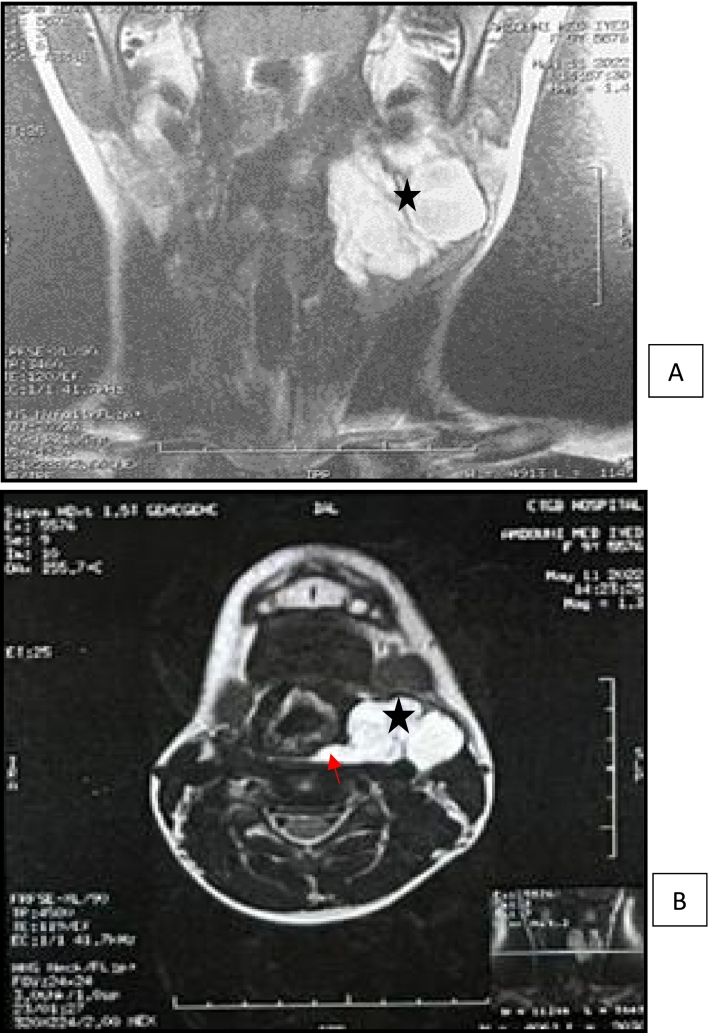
Cervical MRI showing a multiloculated left latero-cervical cyst (black star) on hypersignal on coronal T2 images (A) and axial T2-FATSAT images (B), presenting a retro-pharyngeal extension (red arrow) with no fistula. (For interpretation of the references to colour in this figure legend, the reader is referred to the web version of this article.)
